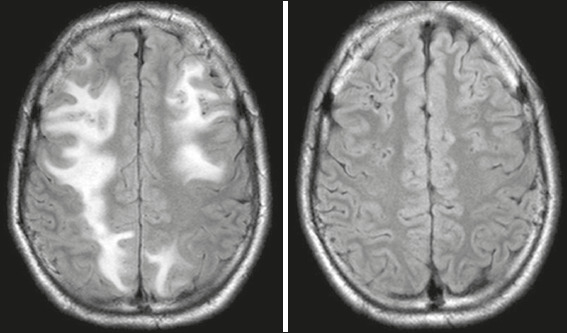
Brain MRI. T2-weighted FLAIR sequence showing the reversibility of lesions.
